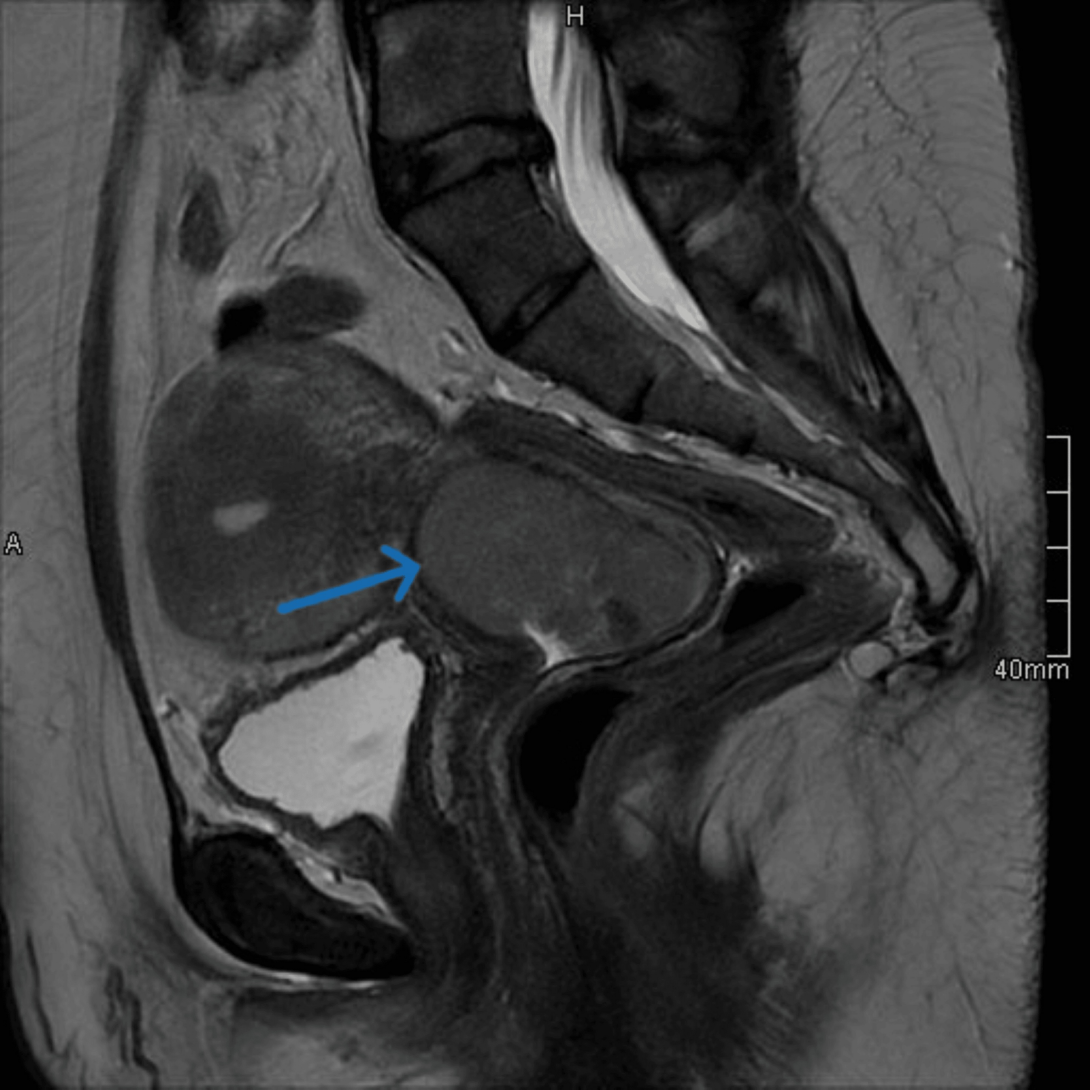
Sagittal T2 MRI of the pelvis demonstrates a large cervical soft tissue mass.
